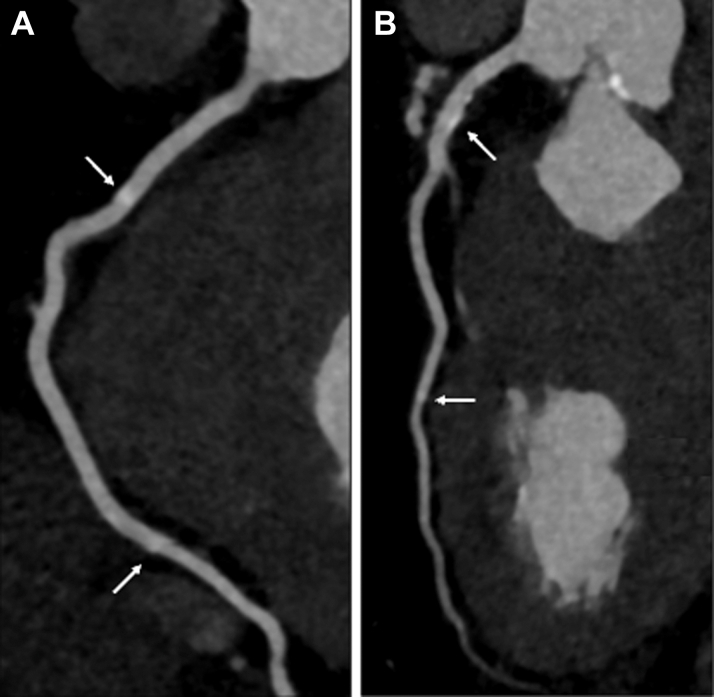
Computed Tomography Coronary Angiography Contrast enhanced computed tomography of the right (A) and left anterior descending (B) coronary arteries revealing radiation-induced calcific atheroma (arrows) and no obstructing coronary artery disease.
